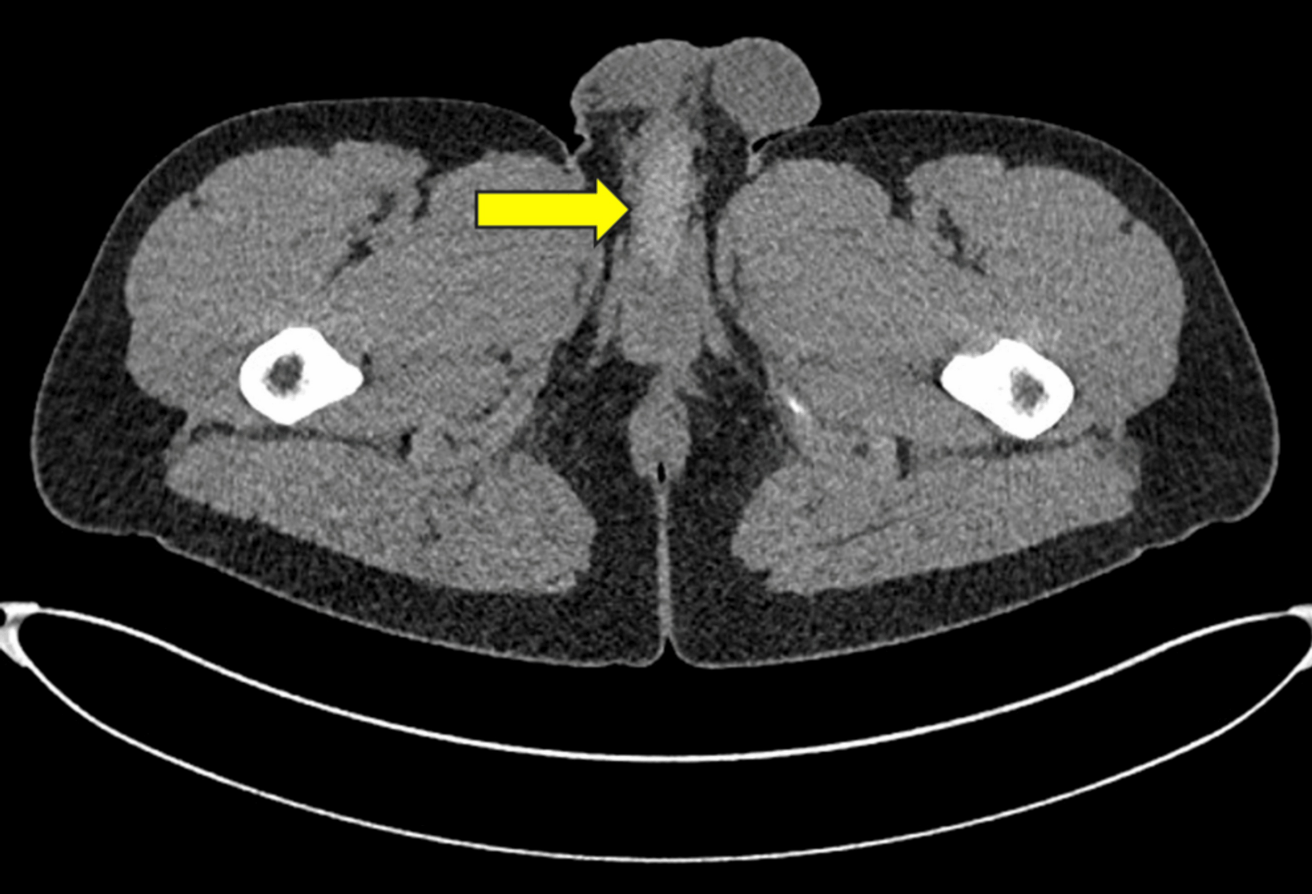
Axial CT image demonstrating a radio-opaque silicone material within the bulbar urethra (yellow arrow).
